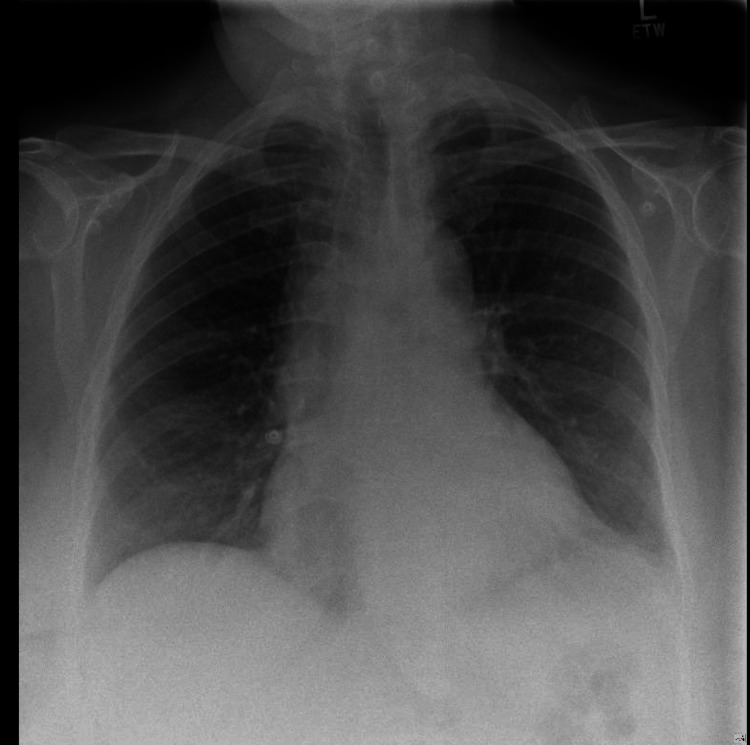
Chest X-ray showing left lower zone airspace opacification with small left pleural effusion
